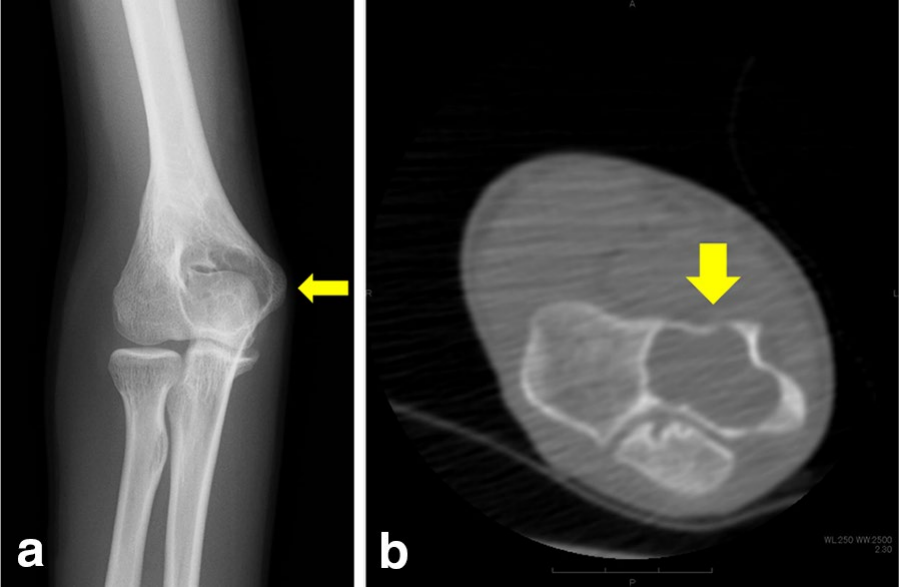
Follow-up plain radiography and computed tomography (CT) findings. At 1 year after excisional biopsy, plain radiography (a) and CT (b) show a large bone cyst in the distal humeral epiphysis (*yellow arrow*).
